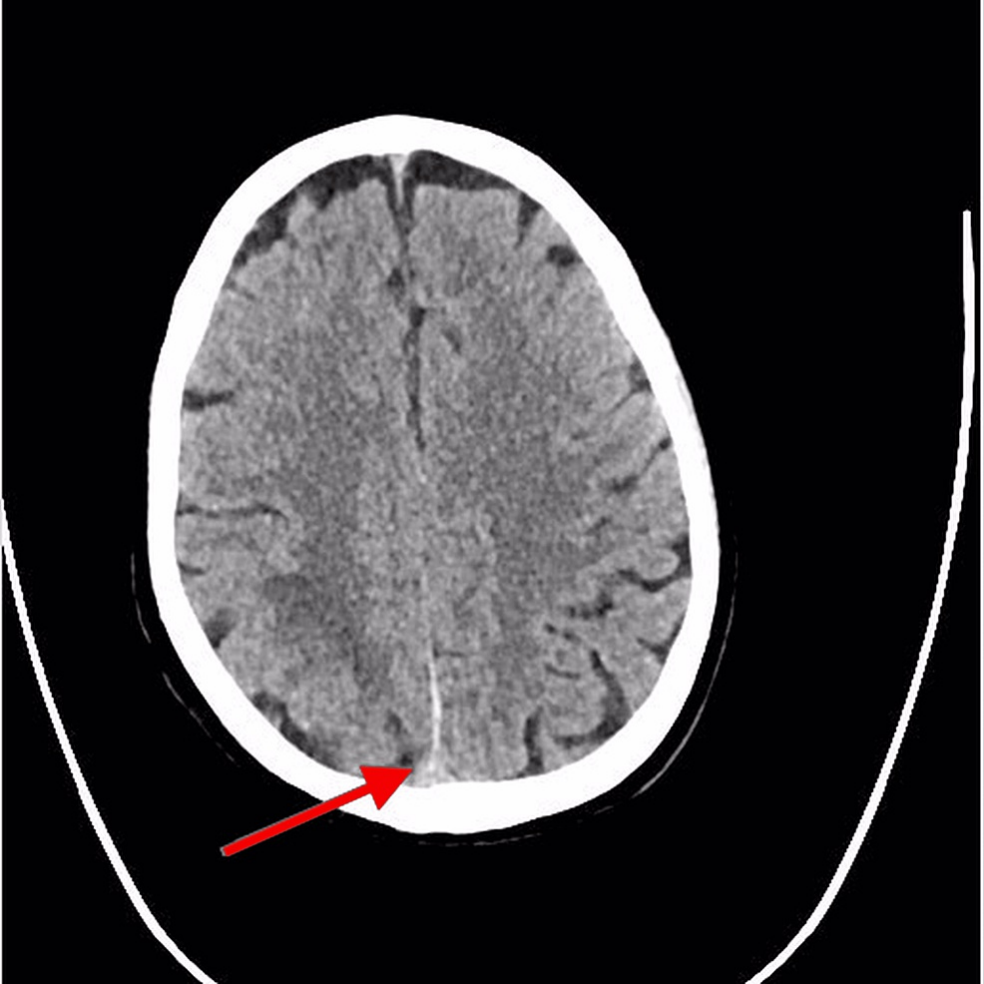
Follow-up plain CT brain (axial section), red arrow pointing towards the superior sagittal sinus showing resolution of the hyperdensity.
